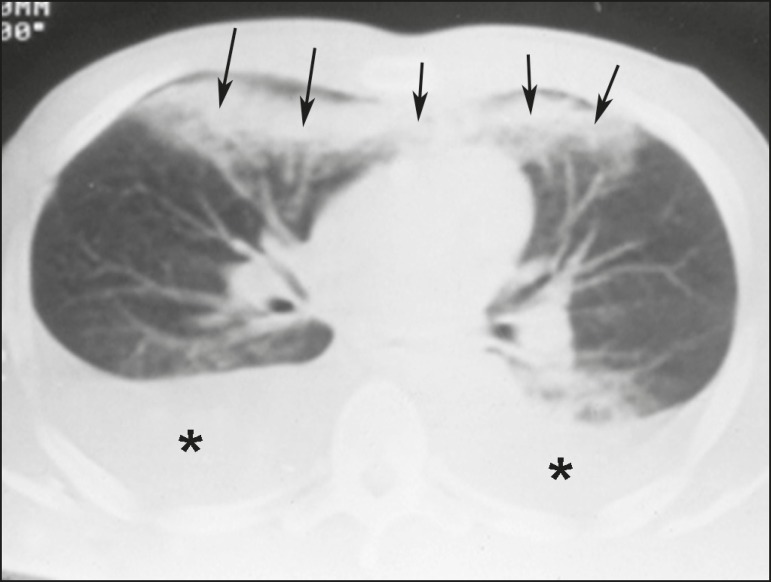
A 26-year-old male gunshot victim. Axial CT scan of the chest
